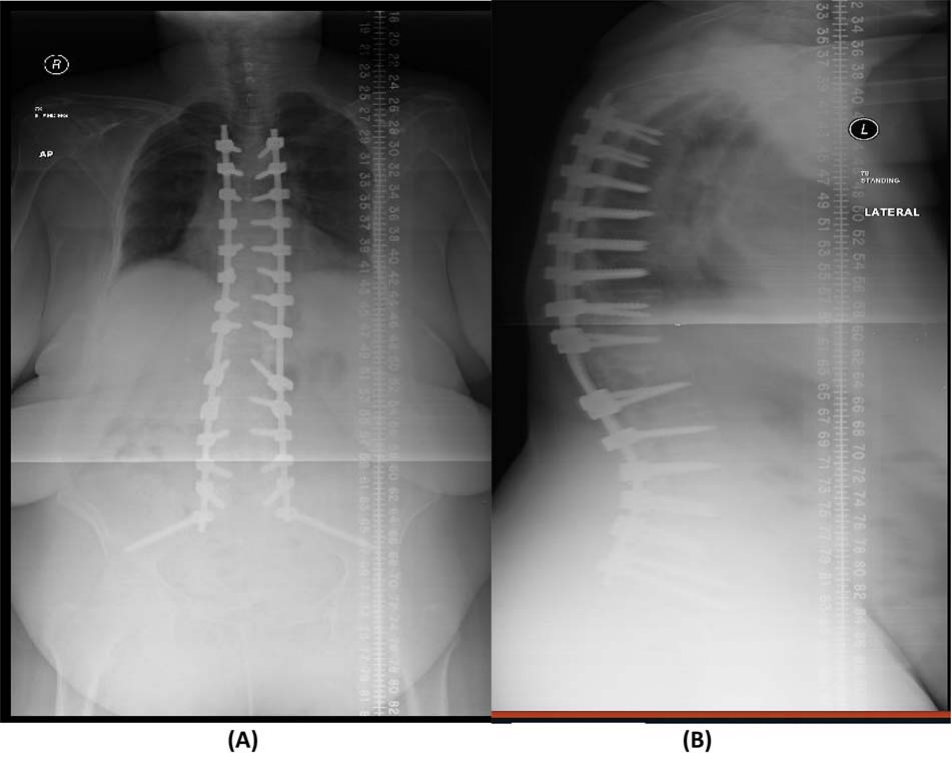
(A) AP lumbar spine X-ray demonstrating final fixation from T5-S2-alar-iliac screws. (B) Lateral spine X-ray.
